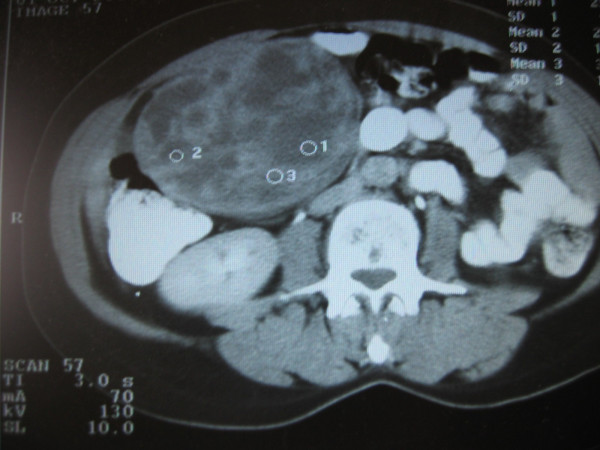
Contrast enhanced CT scan showing heterogeneously enhanced mass located above right kidney.
